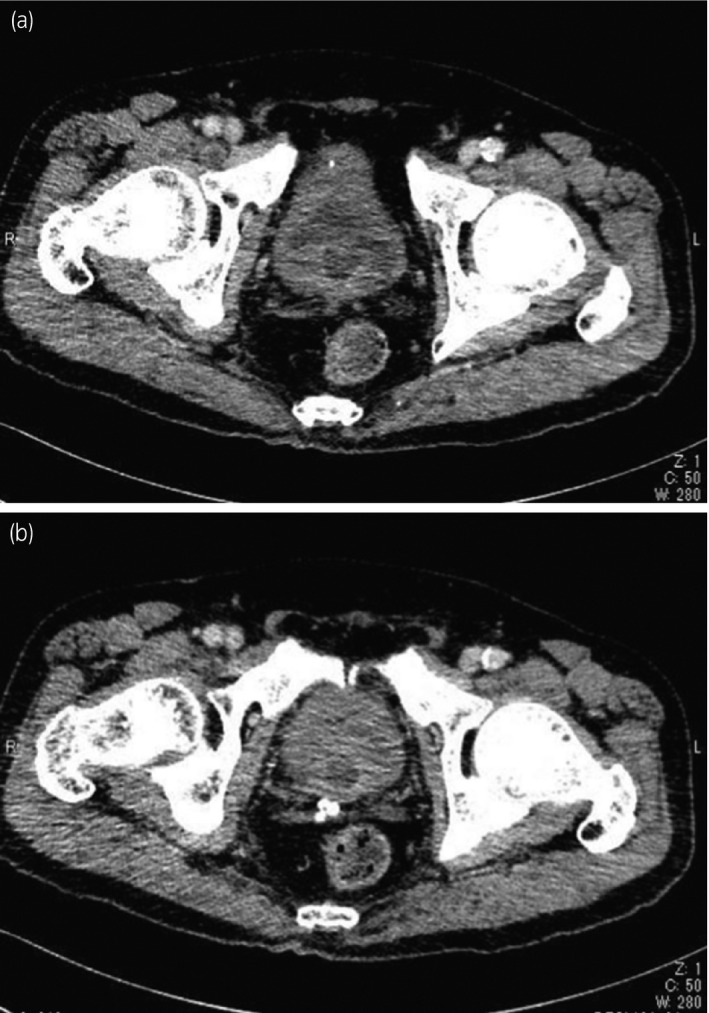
Post‐HoLEP computed tomography scan. Mid‐lobe of the prostate was well enucleated. (b) Sufficient lumen of the prostatic urethra was observed. Neither extra‐prostatic invasion lymph node metastases were detected.
